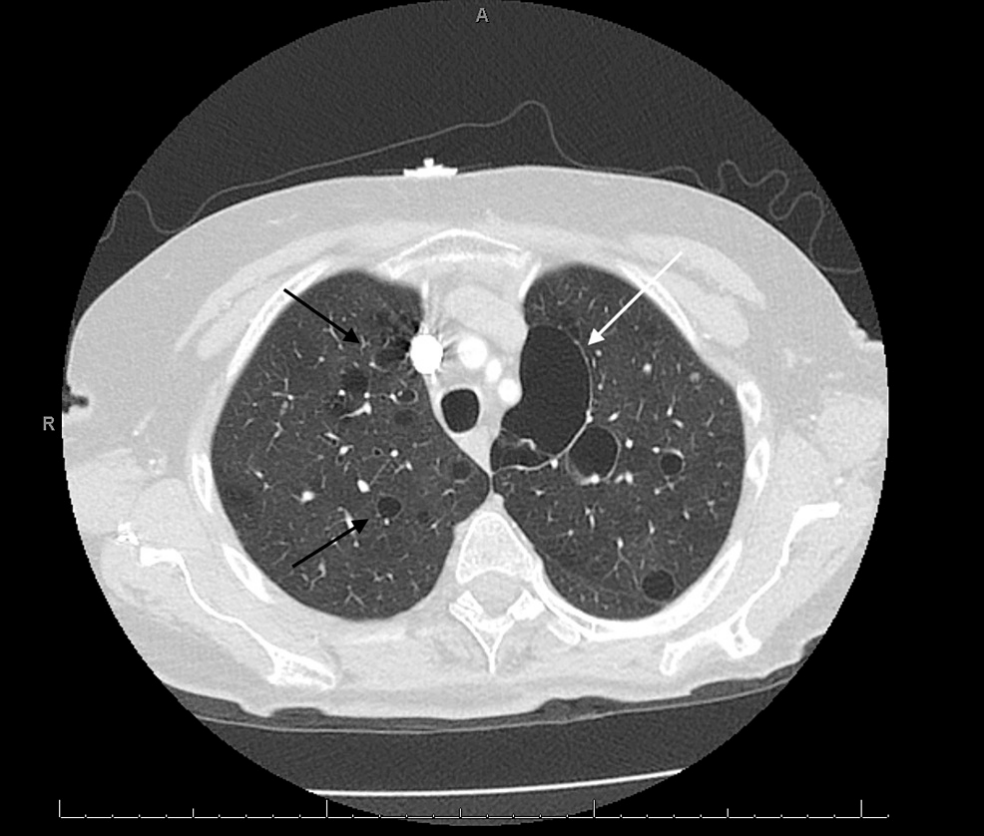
CT scan of the chest showing multiple nodular lesions in both lung fields (black arrows) and a large cystic mass in the left lung field (white arrow)
